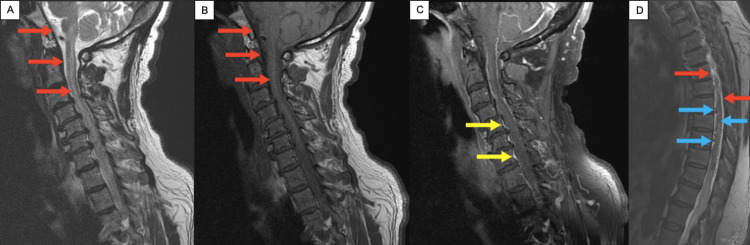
Progressive extension of intracranial hemorrhage into thoracic spine on MRIs The cervical (A, B, C) and thoracic spine (D) MRIs demonstrate the progressive extension of the hemorrhage down the spine. (A) is a sagittal T2-weighted image and (B) is a sagittal T1-weighted image of the cervical spine, with the red arrows demonstrating hypertense signal enhancements along the dural surfaces and intramedullary, thought to correspond to areas of blood. (C) is a sagittal T1-weighted image with gadolinium of the cervical spine, with the yellow arrows demonstrating contrast leaking into the spine. (D) is a sagittal T2-weighted image of the thoracic spine, with the red arrows further demonstrating hypertense signal enhancements along the dural surfaces and intramedullary. Here, the blue arrows also demonstrate serpiginous flow voids intradullary, most prominent seen from T5-10.
